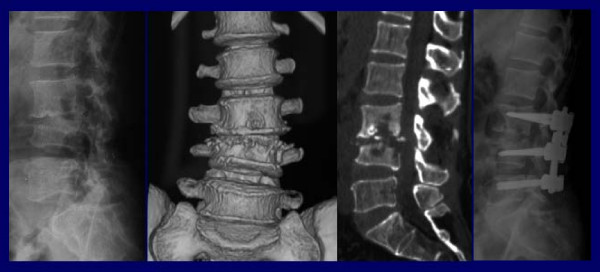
A) Lateral graphy, B) Coronal 3D reconstruction computed tomography, C) Sagittal reformate image: height loss of L4 vertebrae, narrowing disc space with end plates destruction. D) Postoperative lateral graphy: improvement of vertebral axis and disc space.
